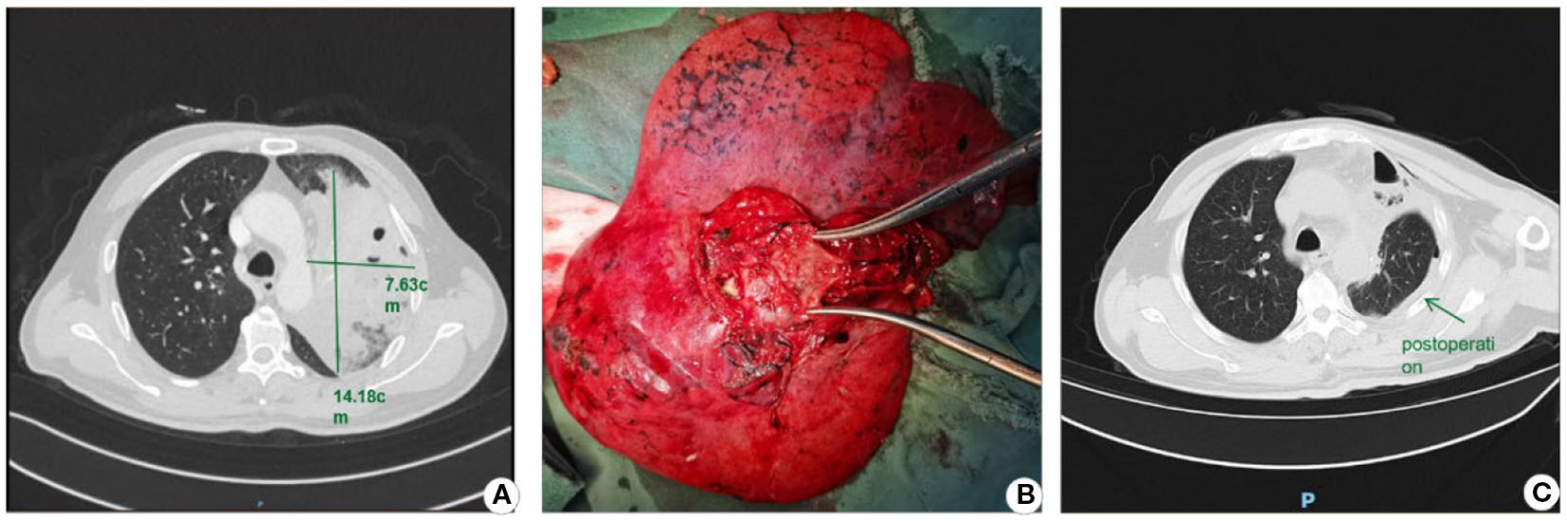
Lobectomy for lung cancer-associated lung abscess. (A) Preoperative CT image; (B) Resected lobe demonstrating the abscess cavity upon dissection; (C) Postoperative CT image.
